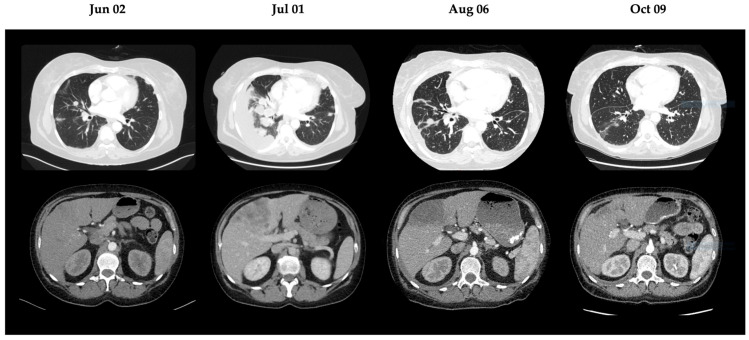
Radiologic evolution of metastatic disease. Serial CT scans of the thorax (top) and abdomen (bottom) demonstrate extensive progression of pulmonary and hepatic metastases on 1 July 2025, followed by rapid regression on 6 July 2025 and 9 October 2025 after initiation of dual targeted therapy. CT: computed tomography.
